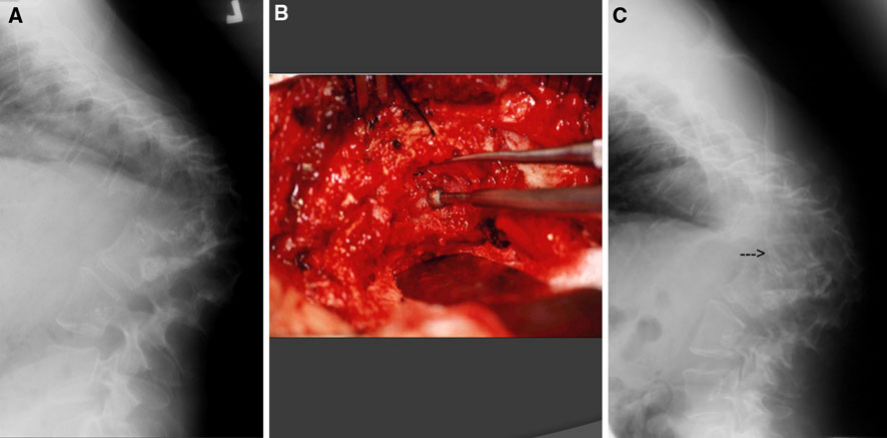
a Severe post-tubercular kyphosis, b internal kyphectomy for spinal cord (*arrow*) decompression, and c post-operative x-ray showed internal kyphectomy and bone graft in position (*arrow*)
